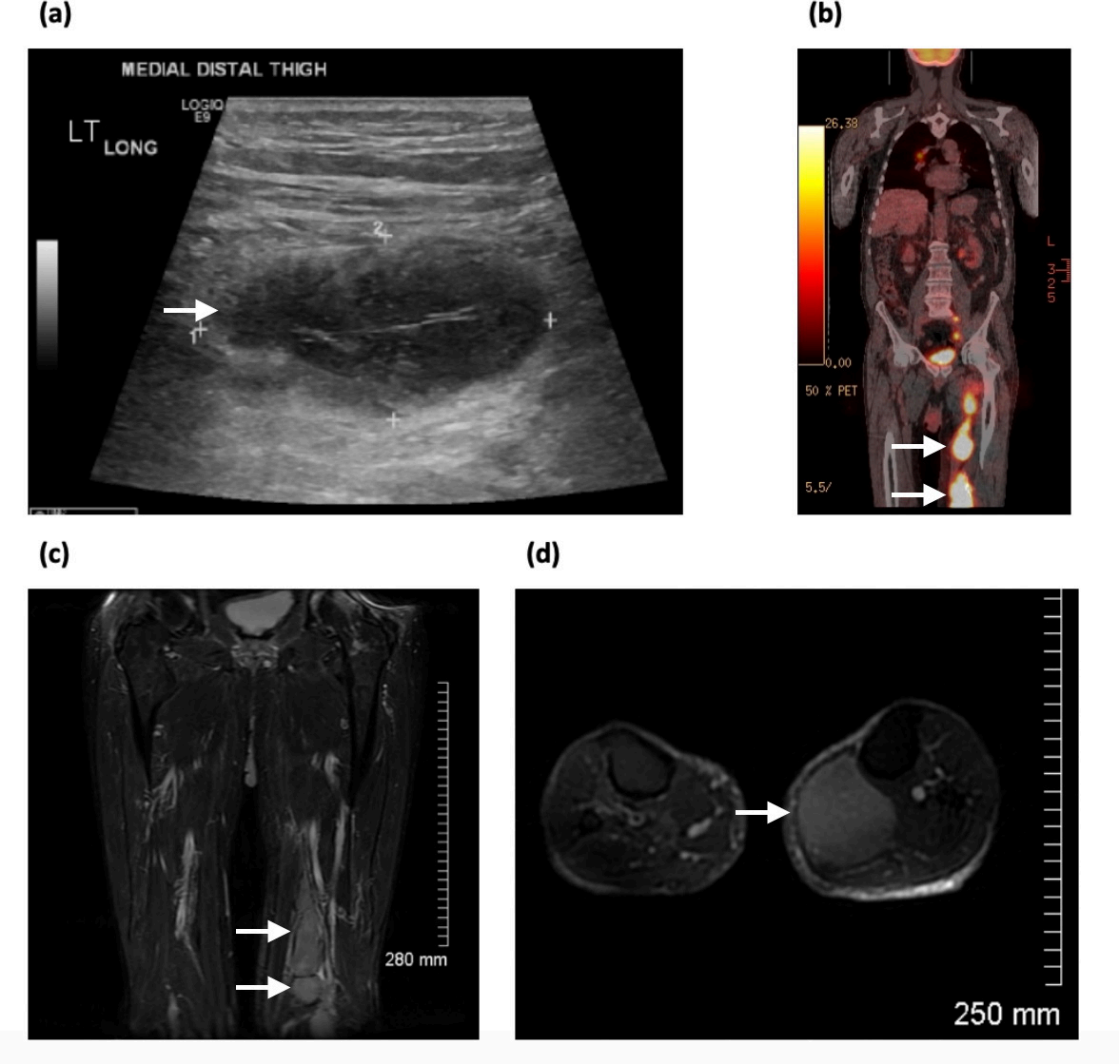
(a) Sonogram demonstrating hypoechoic poorly defined lesion, (b) PET-CT image showing multiple FDG-avid lesions in the medial aspect of the lower limb, (c) coronal *T*_2_ weighted MR image showing multiple hyperintense lesions, (d) axial *T*_2_ weighted MR image demonstrating hyperintensity of the lesions with well-defined margins. FDG, fludeoxyglucose; PET, positron emission tomography.
